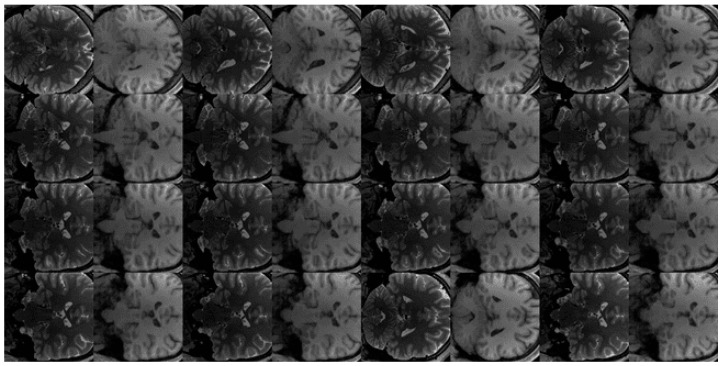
Unpaired CycleGAN synthesis. Example MRI modality translation using unpaired CycleGAN, showing preserved global anatomy and tumour localisation with mild smoothing due to lack of paired supervision.
